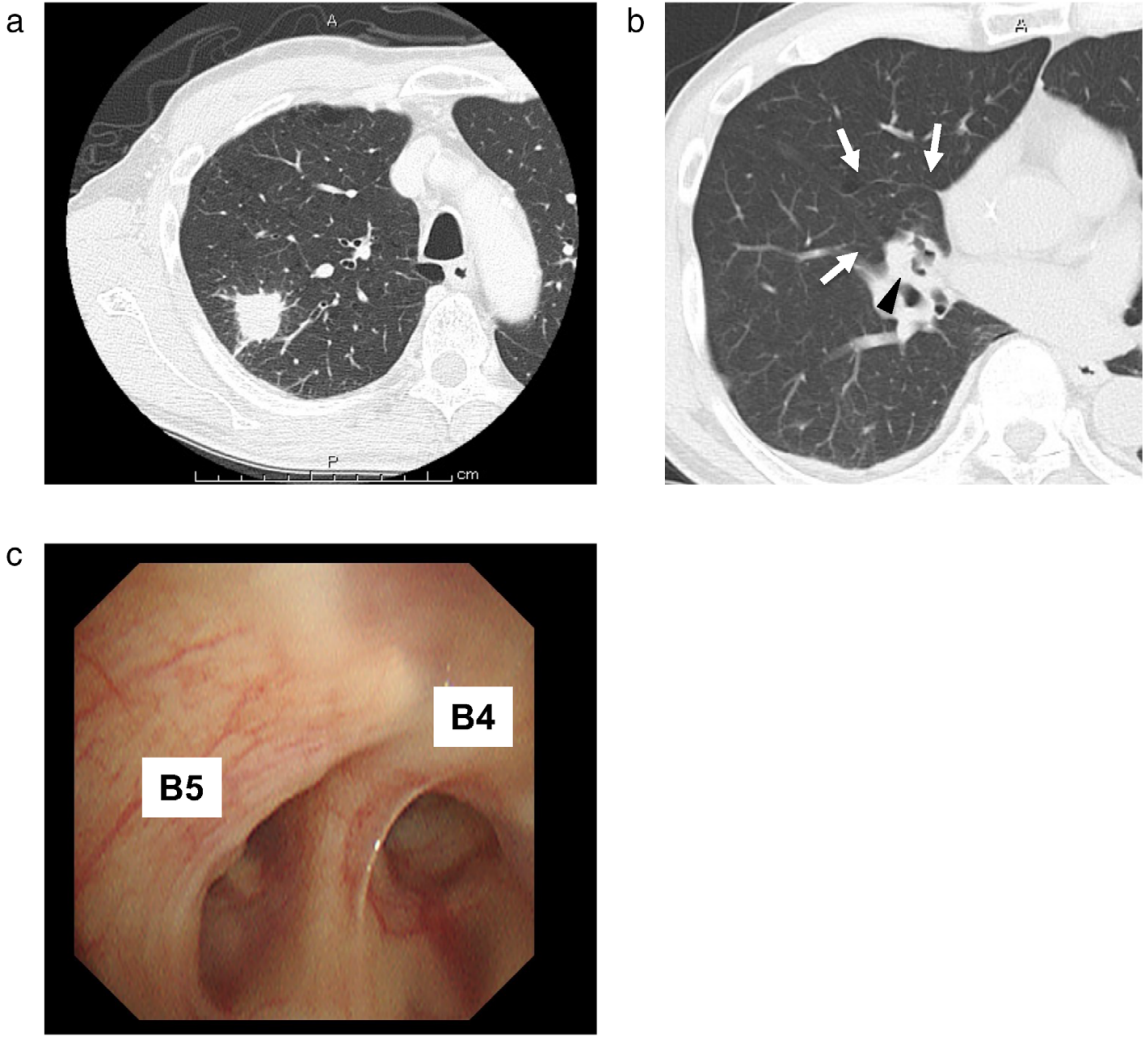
(a) Contrast‐enhanced computed tomography (CE‐CT) showing a 32 mm irregular nodule in the posterior segment (S2) of the upper lobe of the right lung. (b) A small‐sized right middle lobe is detected on computed tomography (white arrowhead). The arrowhead indicates the atelectatic region. (c) Bronchoscopy revealing no abnormal findings in the bifurcation of the right middle lobe bronchus (B4 and B5)
